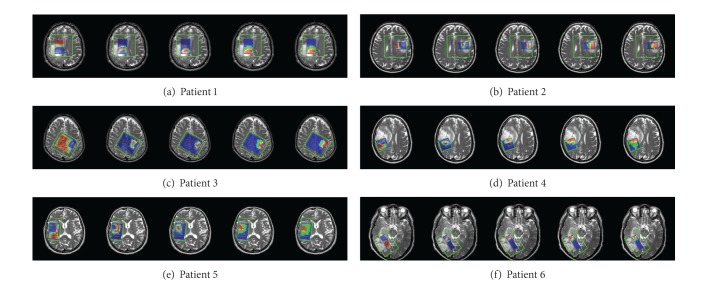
h*-maps and tissue type assignment results compared with expert labeling. All the results are overlaid on the T2-weighted MRI. For each patient, the first 3 images are the *h*-maps for normal, tumor, and necrosis. The fourth image is the assigned tissue types. The last image is the expert labeling.
